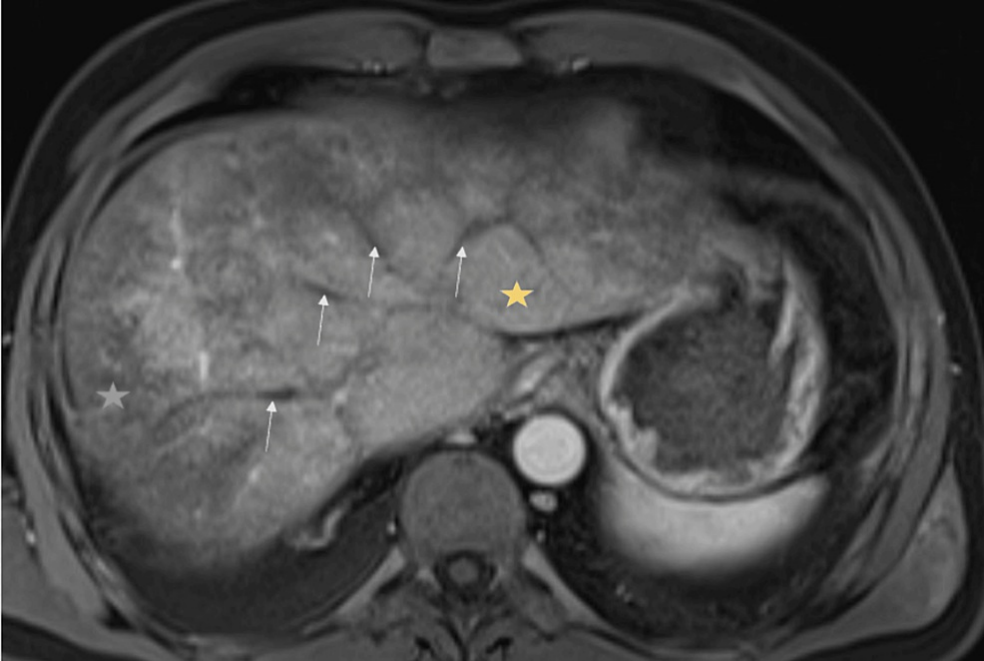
Abdominal MRI post-contrast T1-weighted sequence in venous phase demonstrating findings compatible with Budd-Chiari syndrome. The MRI shows a mottled pattern, with areas of early uptake in the caudate lobe (marked with a yellow star) that coexist with areas of more peripheral uptake (blue star), associated with the absence of opacification of the suprahepatic veins (marked with white arrows).
